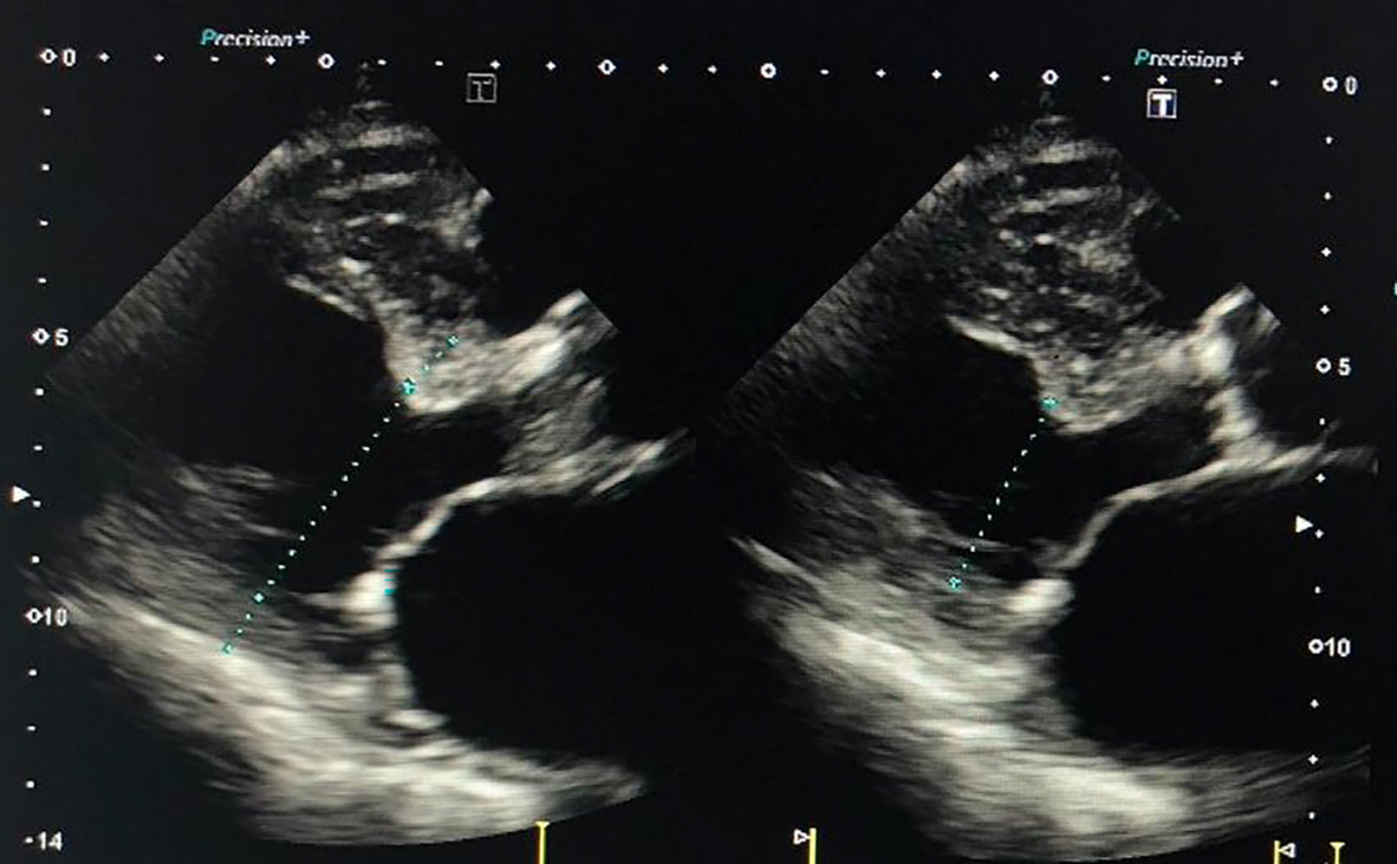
Transthoracic echocardiography. On transthoracic echocardiography, left ventricular end‐diastolic dimension/end‐systolic dimension was 46/36 mm. Diffuse moderate systolic dysfunction of ventricular wall motion was noted.
